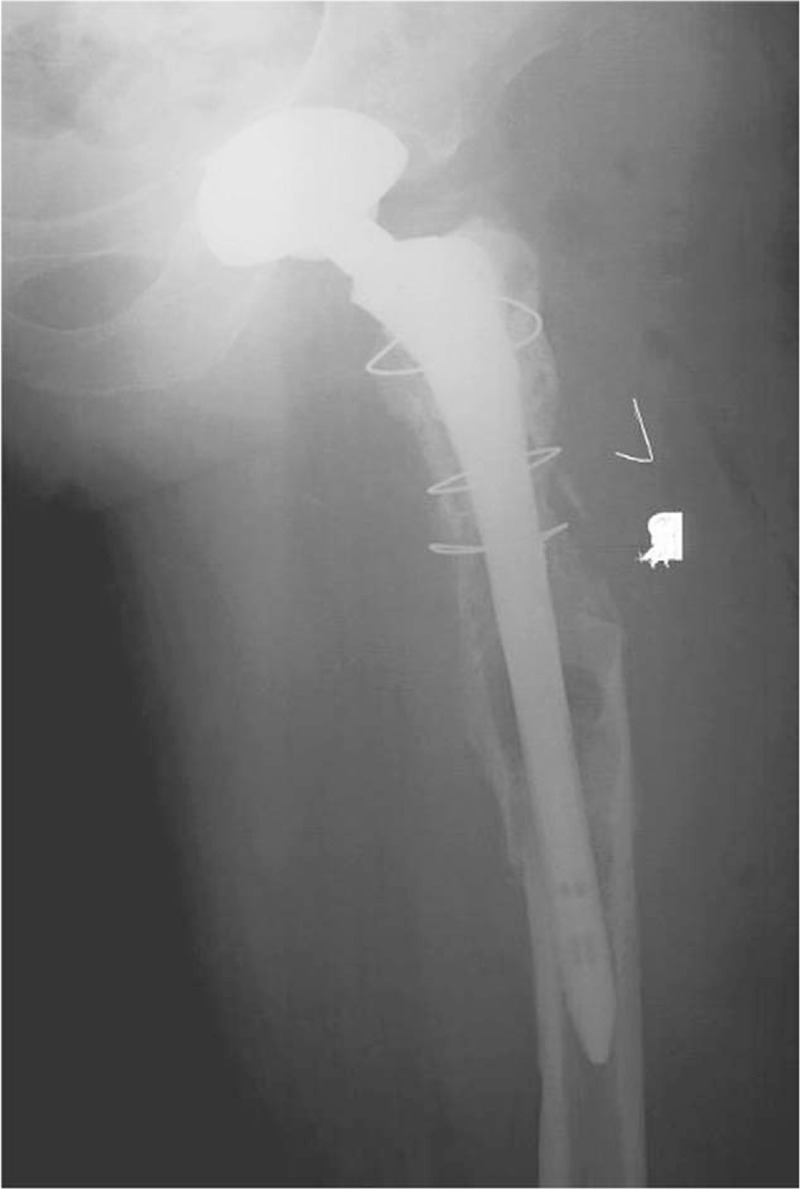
X-ray image after the second RTHA on the left side. RTHA = revision total hip arthroplasty.
